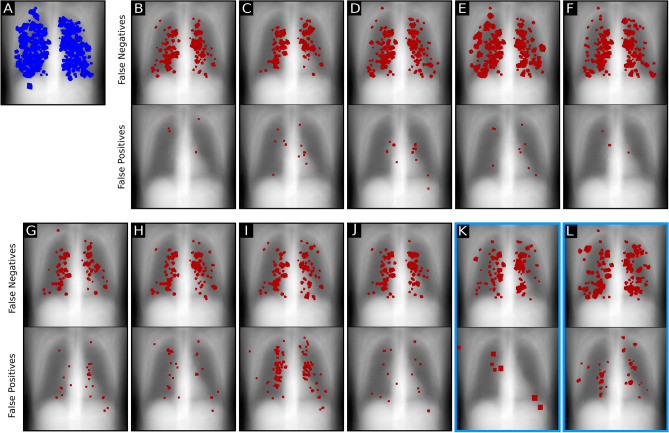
Localization of false negative and false positive predictions in the reader study. Backgrounds were determined by averaging over all reader study radiographs. (A) All inserted nodules of different sizes in all radiographs marked blue. (B–J) False negative and false positive predictions by reader. (K) Location of false negative predictions of RetinaNet and false positive predictions of RetinaNet. (L) False negative predictions of U-Net and false positive predictions of U-Net.
